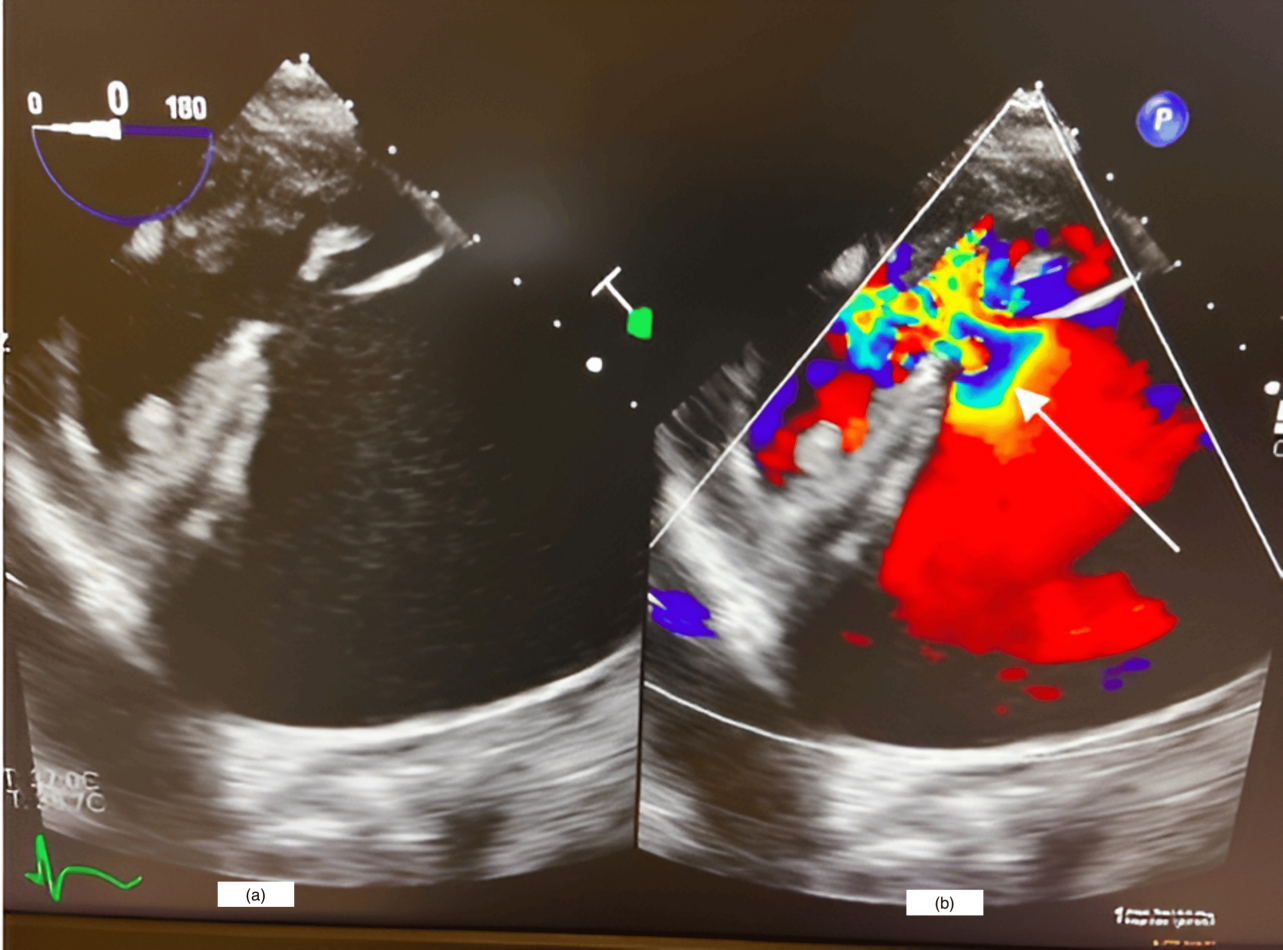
Intraoperative transesophageal echocardiography image showing left ventricular pseudoaneurysm
